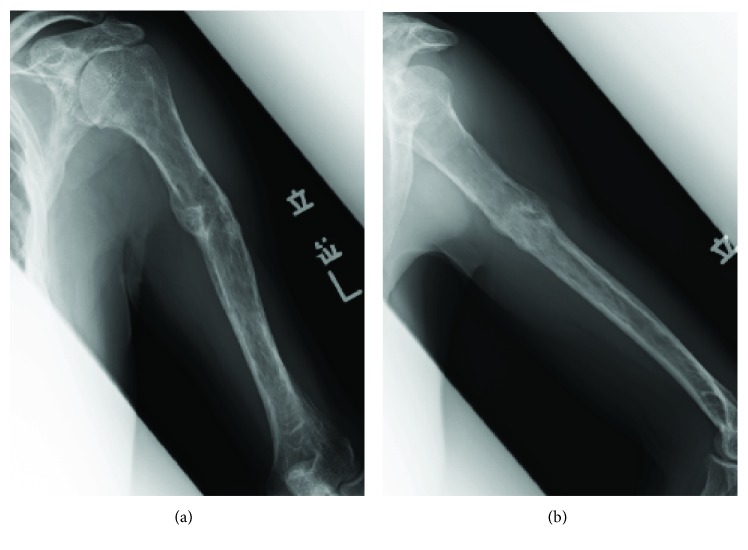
Preoperative radiograph of the left humerus ((a) anteroposterior view; (b) lateral view) showing varus deformity at the healed fracture site and osteolytic lesion with endosteal scalloping at the diaphysis.
